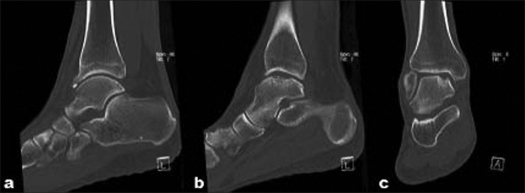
(a, b) Sagittal and (c) coronal CT scan of the right ankle showing osteophytes, a large medial talar dome osteochondral defect, and multiple loose bodies
